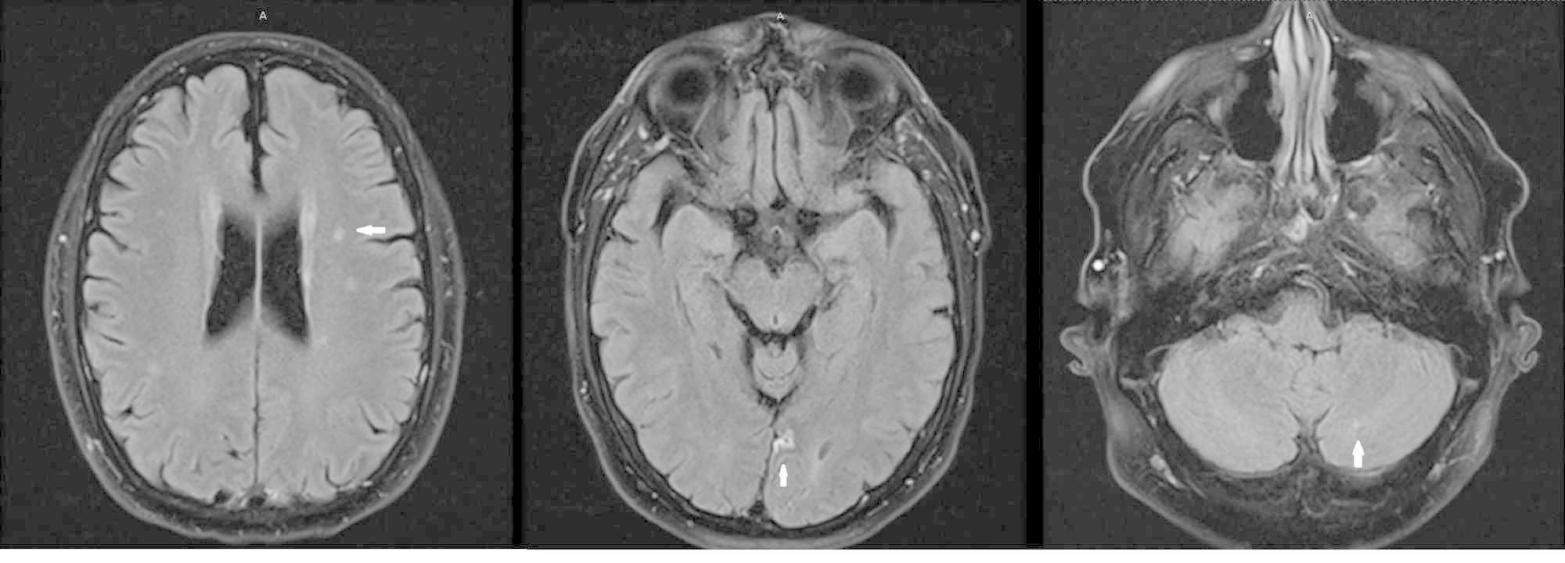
Brain MRI without contrast showing acute ischemic infarcts in the left thalamus (extreme left), the medial occipital lobe (middle), and the cerebellum (extreme right)
